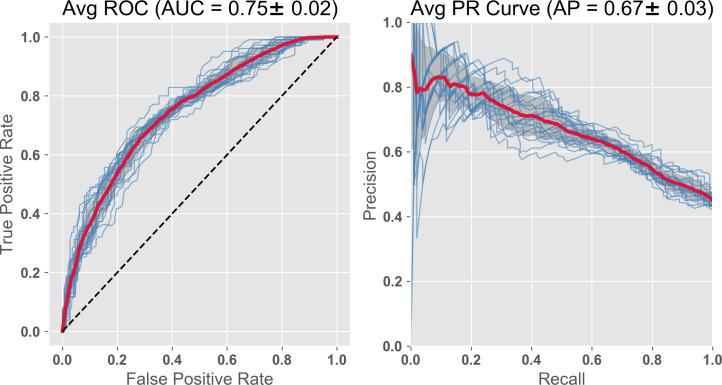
RF Classifier with subset of plasma BMs, compressed cognitive scores and MRI, and demographics. The features include the 2 compressed ADNI cognitive and MRI variables, 14 plasma analytes chosen from the literature and demographics. AUC = 0.75, PR curve = 0.67. This analysis includes all the features used in both Figs 4 and 5 resulting in a slight improvement for both analysis parameters.
